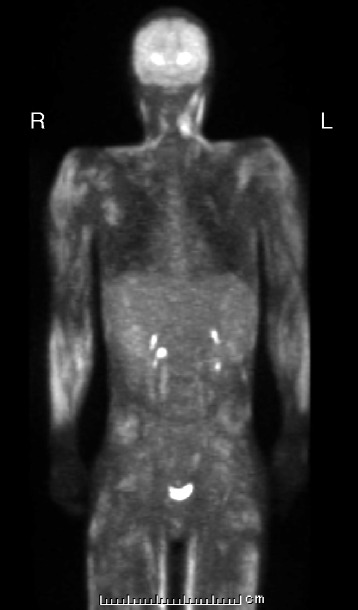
A positron emission tomography-computed tomography examination at the onset of dermatomyositis and hemophagocytic lymphohistiocytosis. No mass lesions was detected
